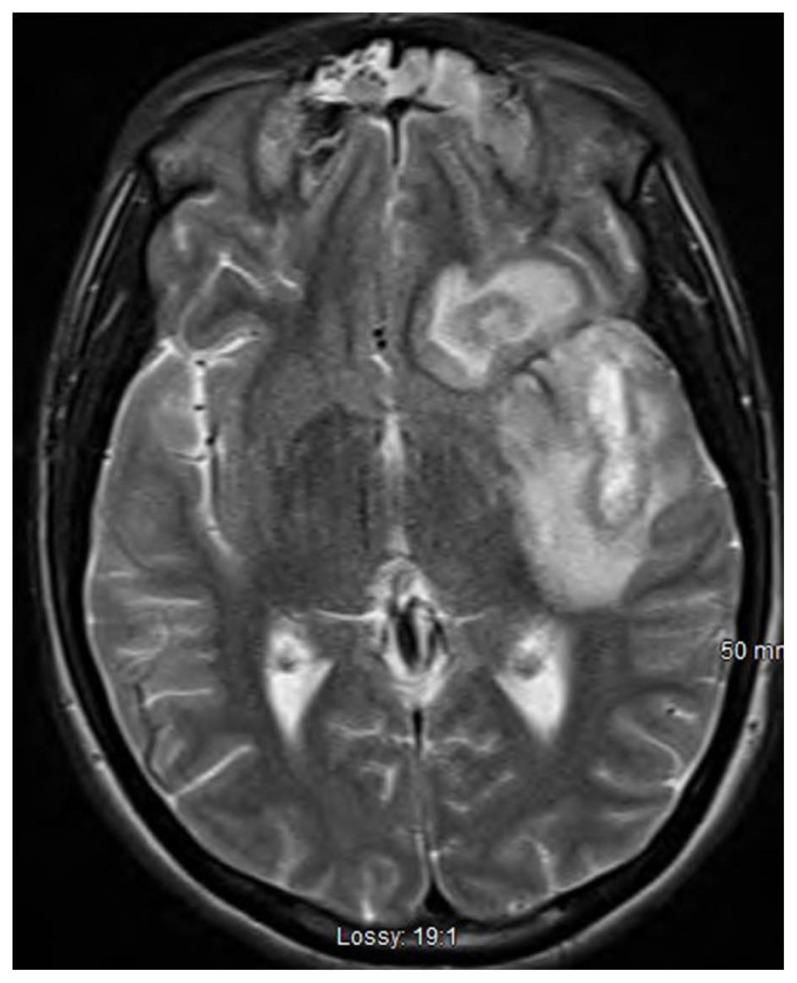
MRI scan of the brain, with gadolinium, demonstrated multilocular fluid collections with enhancing margins in the left temporal lobe and posterior inferior left frontal lobe, and a subdural fluid collection in the anterior and inferior left cranial fossa.
